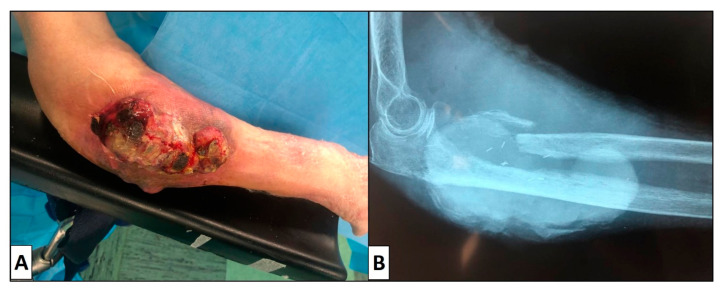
Ulcerated cutaneous lesion (A). Radiographic image (B) shows a big lesion of soft tissue that has infiltrated underlying bone plans, in particular proximal radio, which is almost completely eroded. The proximal ulna also appears infiltrated and partly rare.
